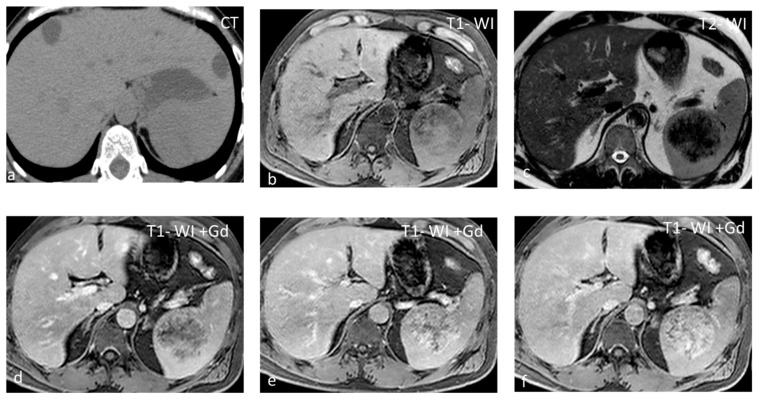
SANT appearing slightly hypodense on non-contrast CT (a) and isointense on T1-WI (b), with small areas of hyperintensity due to hemorrhage. On T2-WI (c), the lesion is heterogeneous, predominantly hypointense, with hyperintense radiating septa. After gadolinium administration, enhancement is progressive (c–f) along the radiating septa converging toward the center of the lesion.
